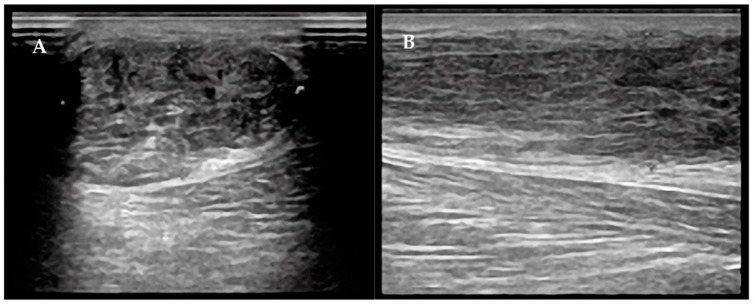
Transverse (A) and longitudinal (B) ultrasonographic images of the Achilles tendon after tenorrhaphy surgery following complete rupture. The loss of the fibrillar structure, the inhomogeneity, and the surgical material in the context of the tendon are "normal" aspects after surgical repair.
